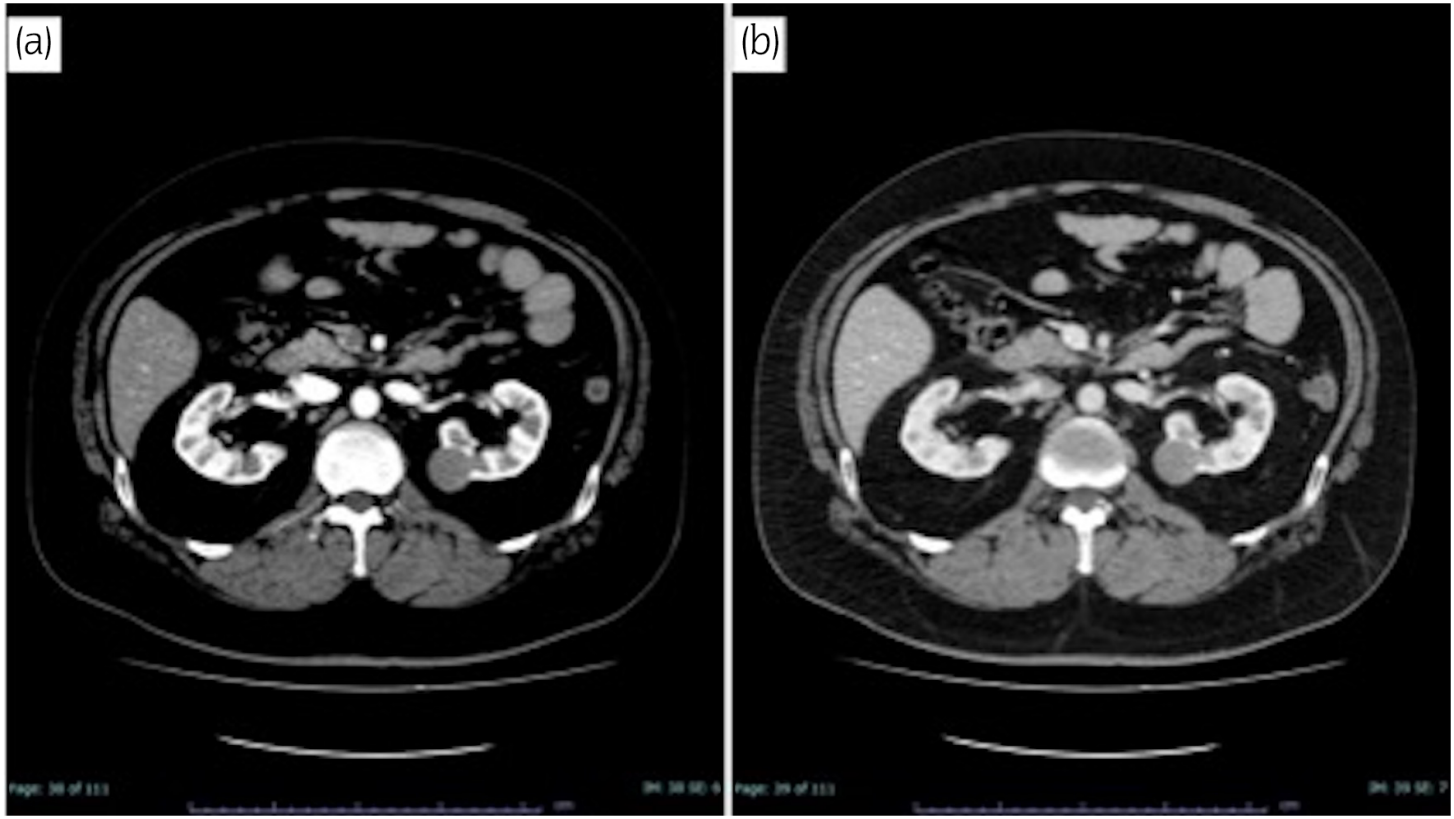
Abdominal dynamic CT. A well‐defined mass with a diameter of 27 mm was seen on the medial dorsal side of the left kidney with a weak contrast effect in the early phase (a) and a progressive increase in contrast effect in the equilibrium phase (b).
